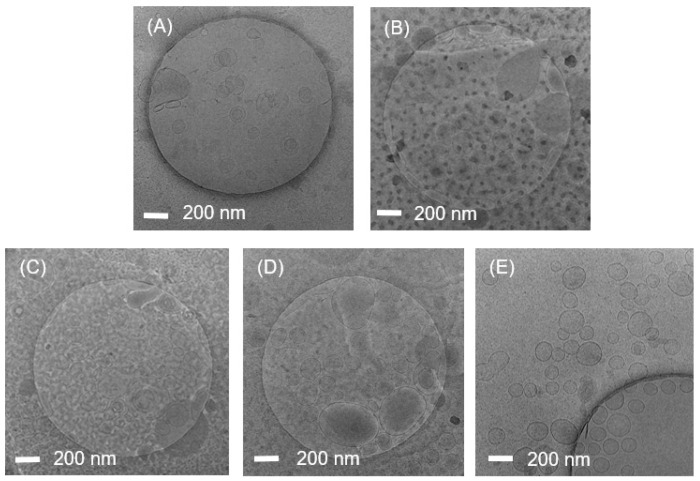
Cryo-EM images of liposome solutions prepared using (A) homo mixer, (B) microfluidizer, (C) bath sonication, (D) horn sonication, (E) focused ultrasonication.
